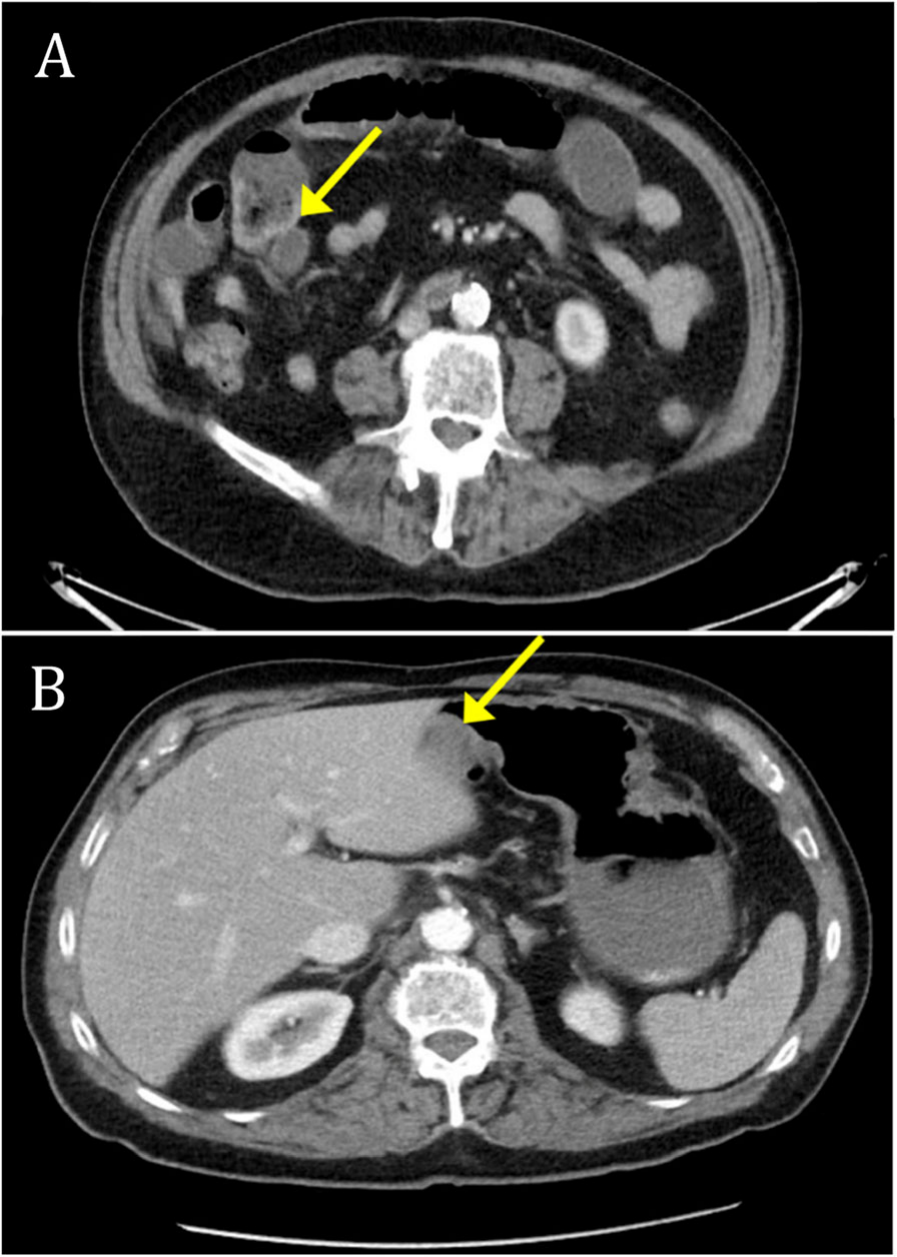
Computed tomography (CT) of the abdomen. Abdominal CT scans of first admission compared with second admission. a Partial bowel obstruction noted on first admission with transition point (*arrow*). b Nodular mural thickening of the anterior aspect of the gastric antrum (*arrow*)
